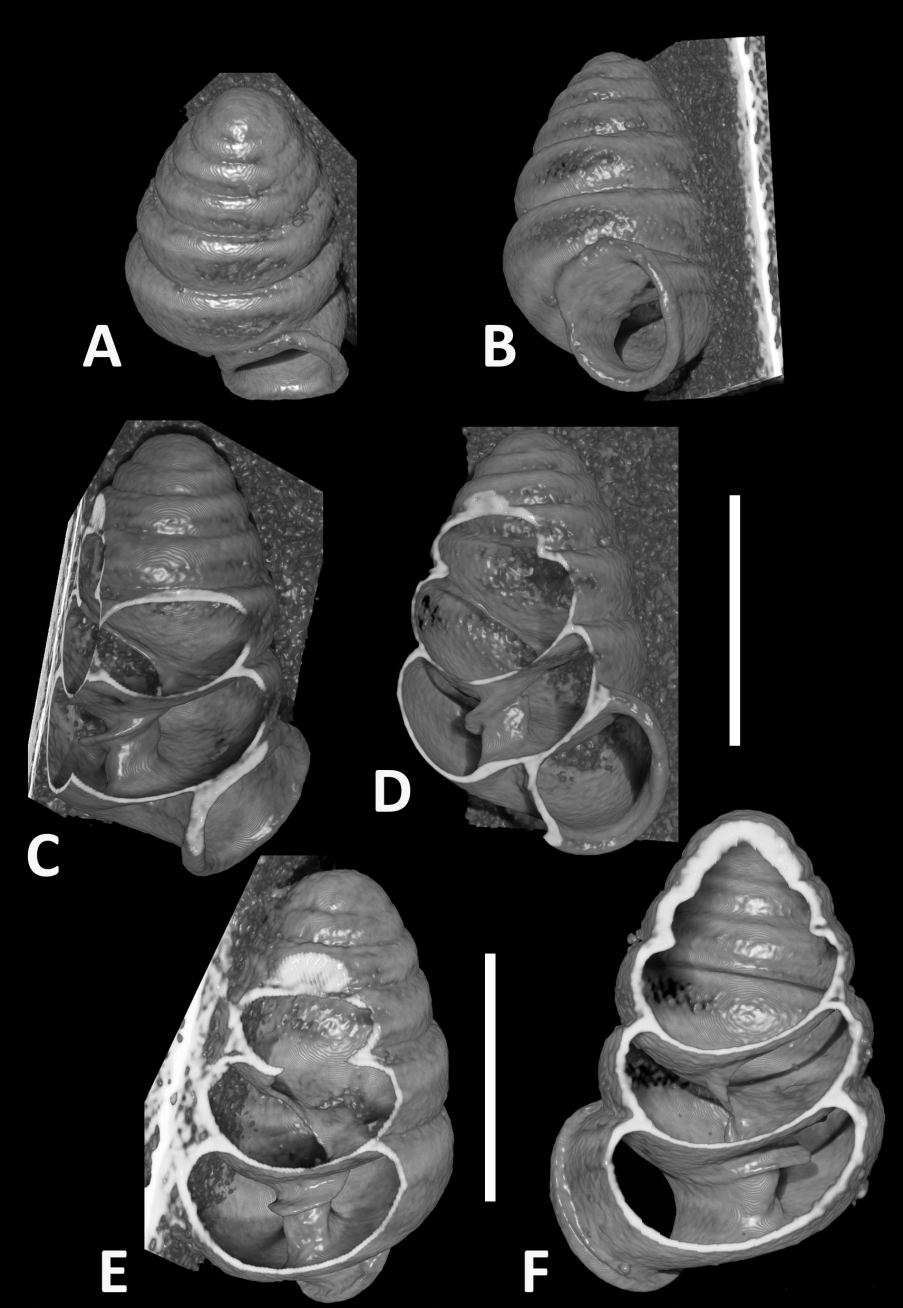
CT images showing columellar apparatus of *Zospeumschaufussi* von Frauenfeld, 1862, lectotype (NHMW 71837). Scale bar: 500 μm.
